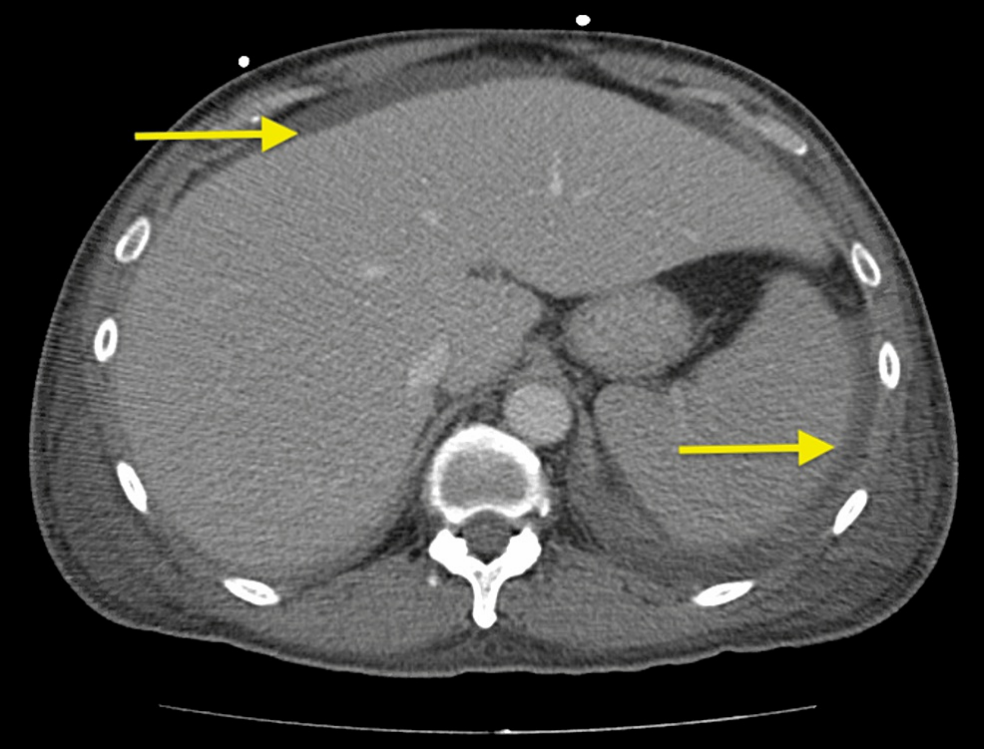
CT abdomen showing hepatosplenomegaly with a small amount of perihepatic and perisplenic free fluid (arrows)
